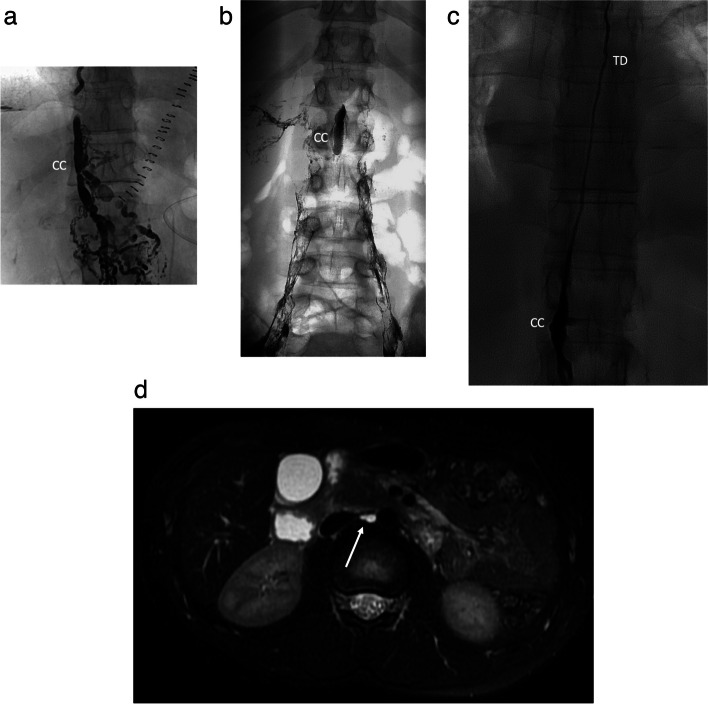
a-d The chyle cistern (CC) (a-c) and thoracic duct (TD) (b, c). The CC is usually located at the level of L1-L2, and it has a linear (a) or oval (b) shape. Sometimes the TD can be seen in T2-weighted sequences on MRI, arrow (d)
